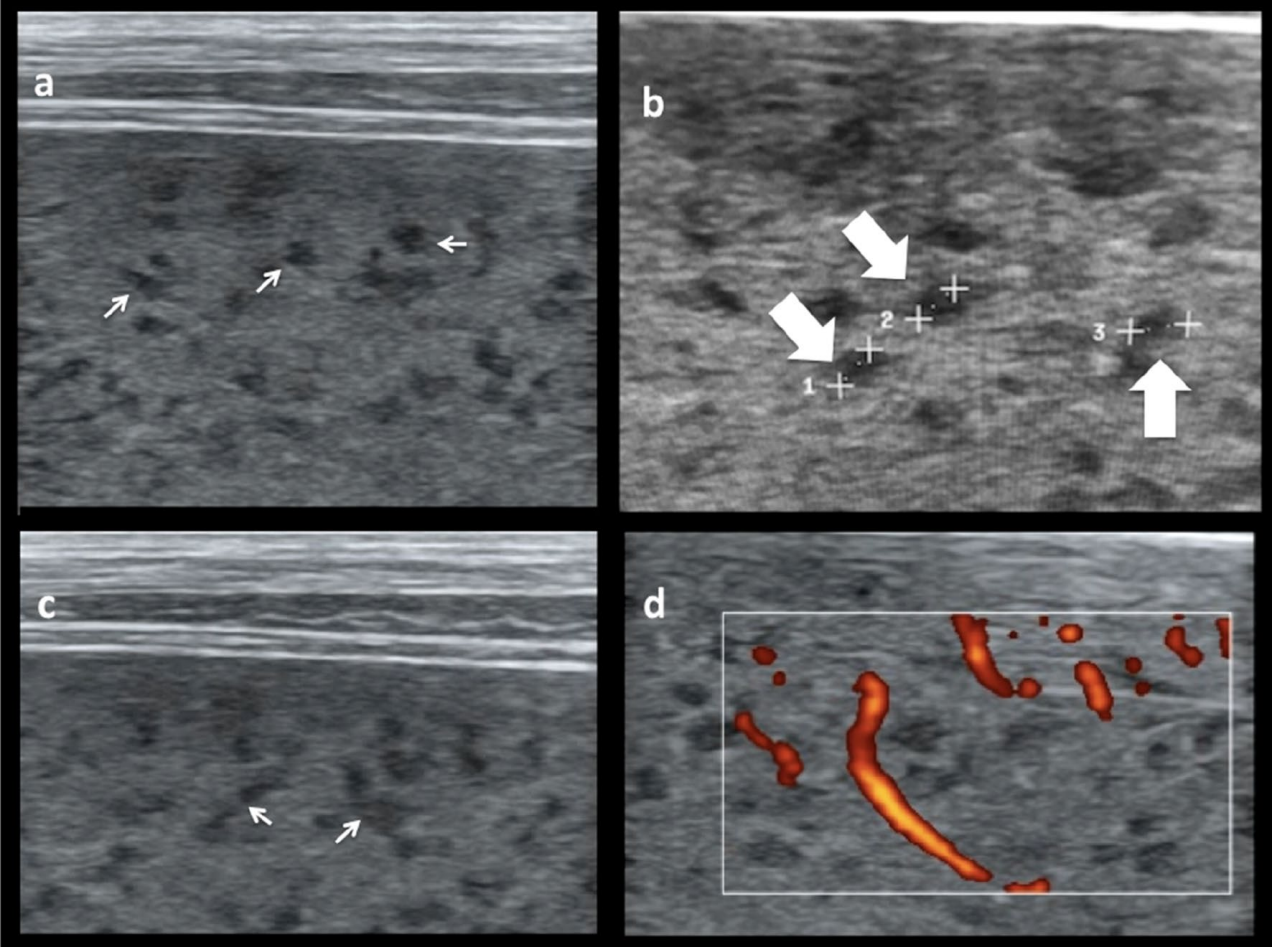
Sonographic appearance of the spleen of index patient. a Numerous tiny hypoechoic areas (arrows) are seen throughout the splenic tissue. b The areas measure about 2 mm in diameter (Block arrows: 1: 1.9 mm, 2: 2.0 mm, 3: 2.4 mm). c In some still views a more linear appearance is noted which is better visible in the moving scan (see Additional file 1: Video clip S1a). d Using the power mode it can be seen that some of the structures follow the vasculature (see Additional file 2: Video clip S1b); others seem isolated
